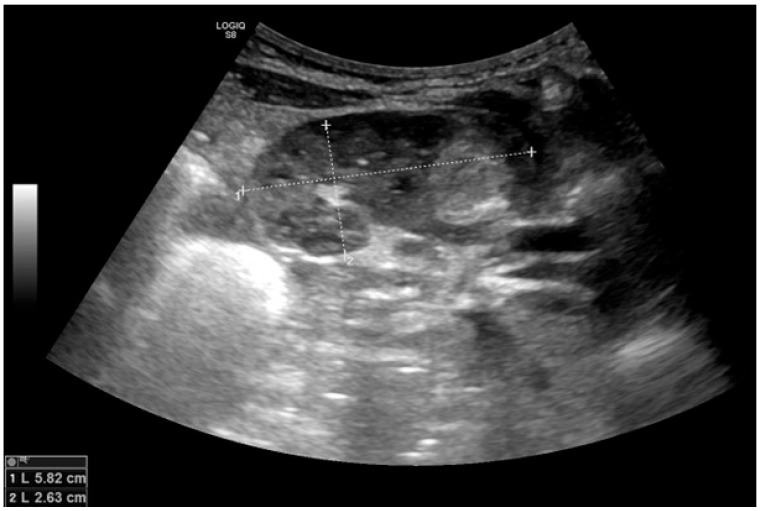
Abdominal ultrasound at diagnosis. Longitudinal sonographic view of the left kidney shows a well-defined, predominantly solid, mildly heterogeneous mass in the upper pole (calipers).
